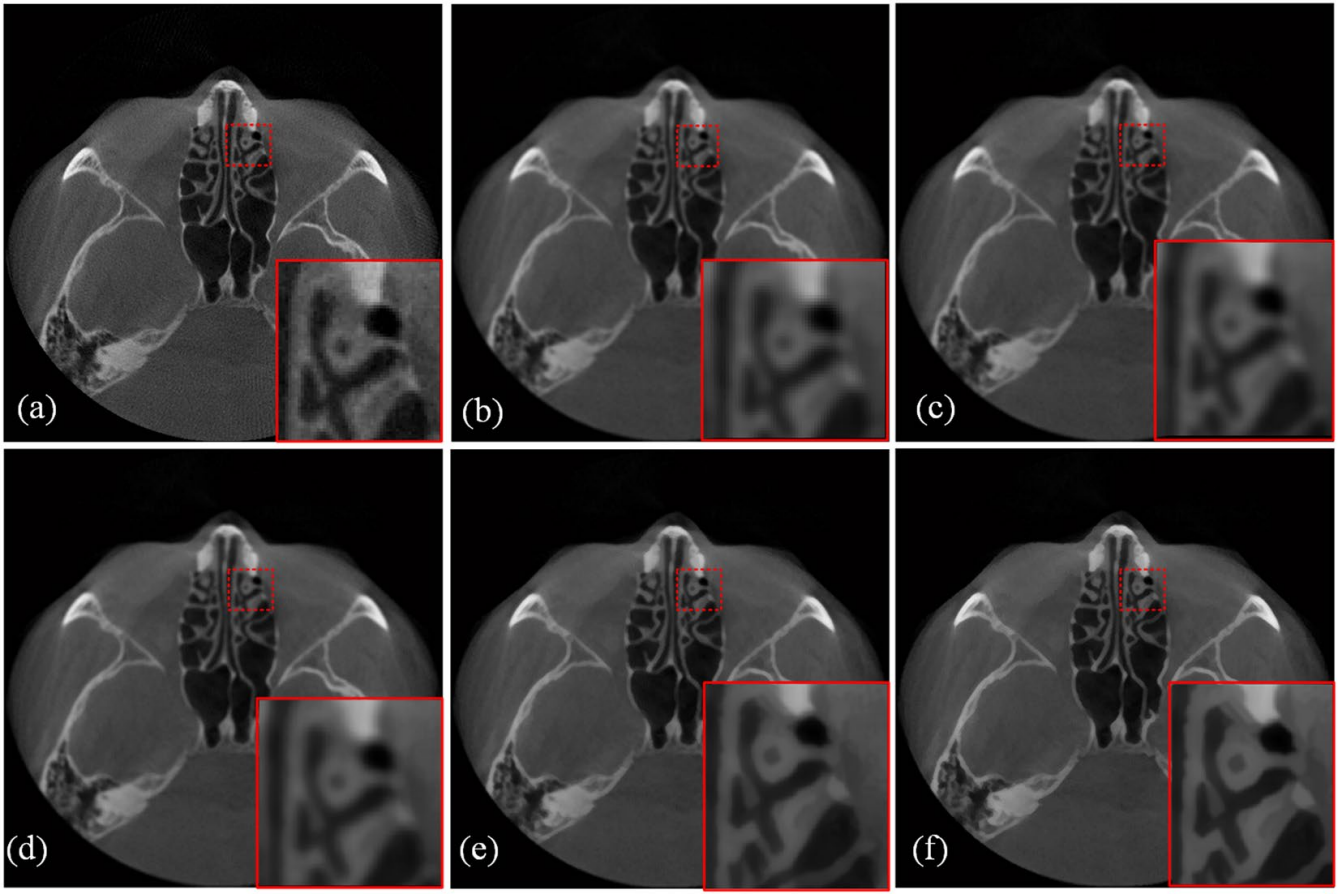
Comparison of SR reconstructed images for another slice of the brain CT images. (a) Original CT image. (b) Input LR CT image downsampled from (a). (c) Reconstructed image obtained using the bicubic interpolation method (PSNR = 33.1202, RMSE = 5.6302). (d) Image reconstructed using the proposed method with 1 iteration (PSNR = 33.2434, RMSE = 5.5509). (e) Image reconstructed using the proposed method with 5 iterations (PSNR = 33.8063, RMSE = 5.2027). (f) Image reconstructed using the proposed method with 7 iterations (PSNR = 33.8809, RMSE = 5.1581). All images are displayed in the same window. This display window is [0, 255].
